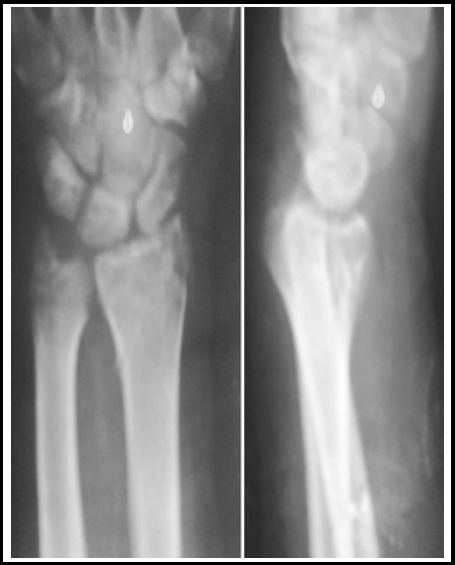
Radiographs after removal of K-Wires showing Fracture Union.
